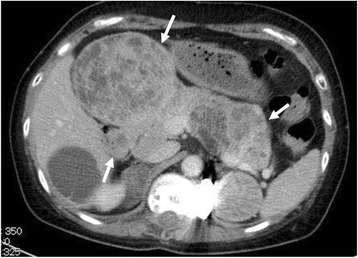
Computed tomography (CT) at 2 months after the second surgery. Axial contrast-enhanced CT reveals multiple metastatic lesions in the pancreas (*arrows*)
